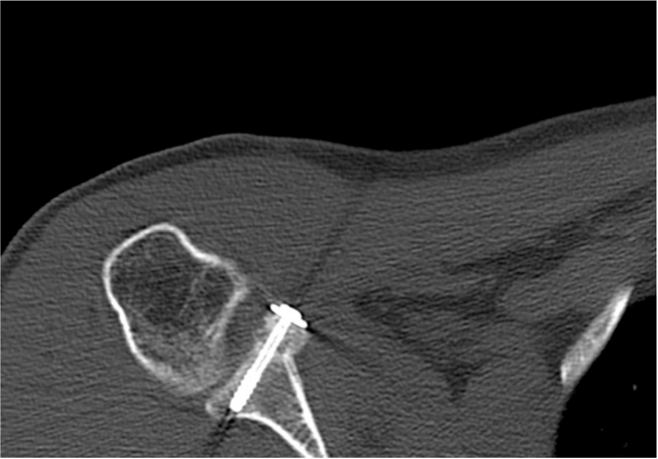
Three-month follow-up computed tomography scan with timely ossification of the attached bone piece.
